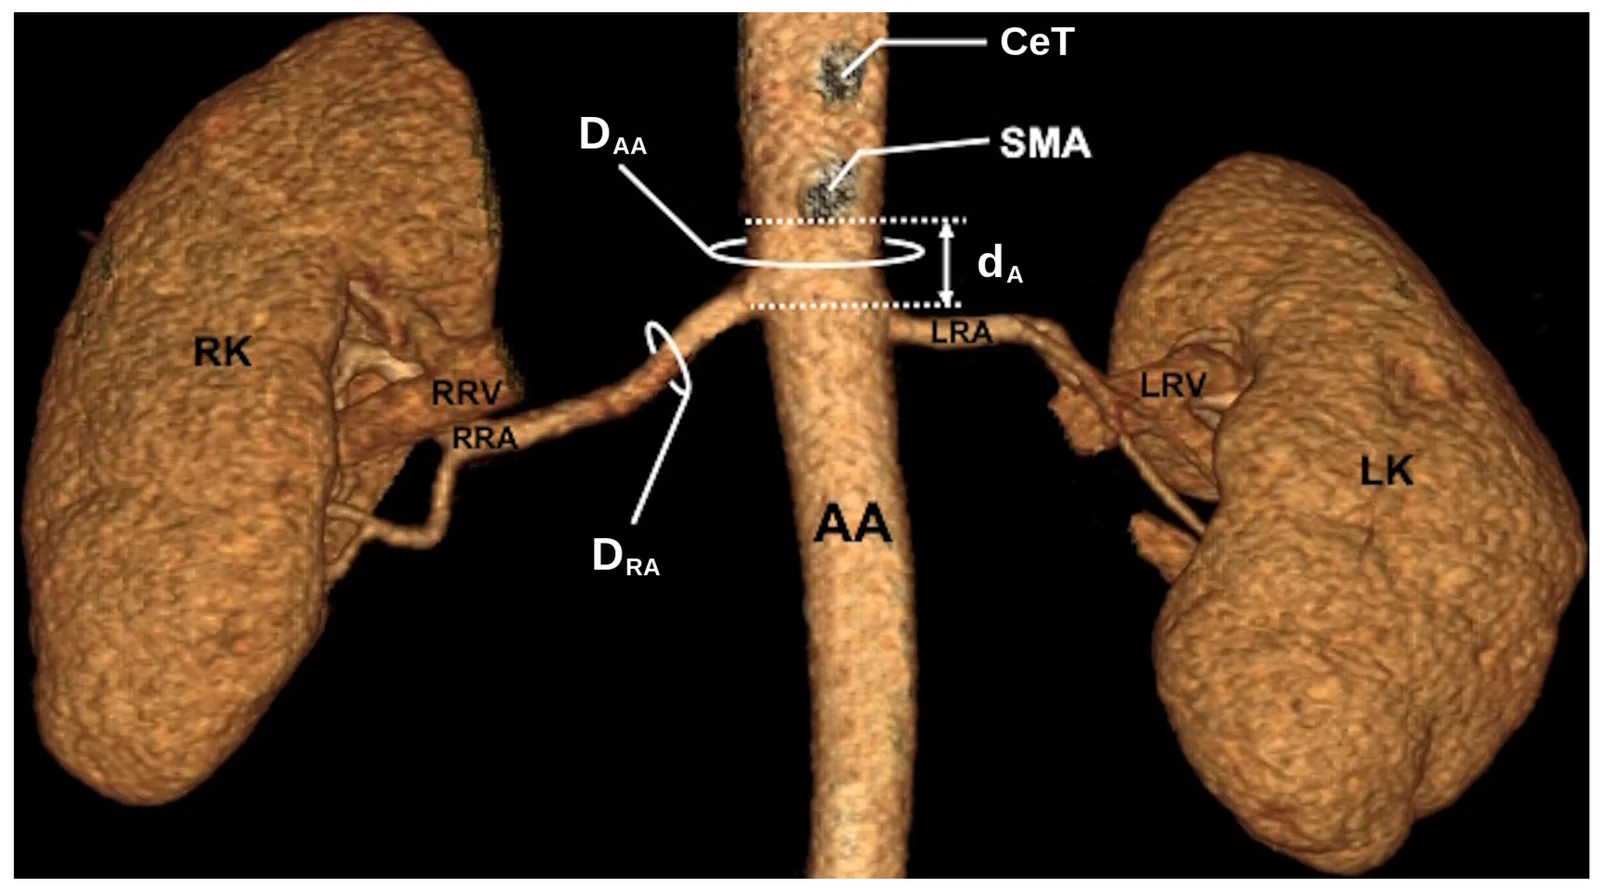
Scheme of the morphometric and topographical measurements, arterial side (three-dimensional reconstruction of contrast-enhanced computed tomography, arterial phase). Aortic diameter (D_AA_) measured 5 mm above the origin of the upper or single artery, renal artery diameter (D_RA_) 15 mm from the ostium, and craniocaudal distance of the origin from the SMA (d_A_). AA, abdominal aorta; SMA, superior mesenteric artery; CeT, coeliac trunk; RK/LK, right/left kidney; RRA/LRA, right/left renal artery; RRV/LRV, right/left renal vein.
